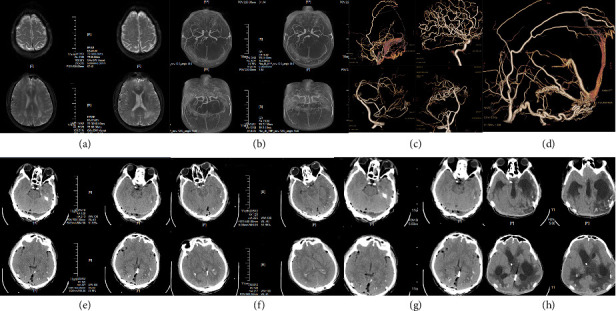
The patient, a 54-year-old male, was admitted to the hospital with a chief complaint of “gradually worsening low mood over the past year, with significant exacerbation in the last two months.” (a, b) Brain imaging revealed multiple abnormal vascular structures, including arteriovenous fistulas, accompanied by white matter edema, congestion, and demyelinating changes. (c, d) Cerebral angiography confirmed the presence of dural arteriovenous fistulas involving the bilateral transverse sinuses, sigmoid sinuses, and the torcular herophili (confluence of sinuses). (e) On the first postoperative day, the patient remained in a drowsy state of consciousness; a follow-up cranial CT scan showed no evidence of intracranial hemorrhage or cerebral infarction. (f) On the first postoperative night, the patient experienced a sudden loss of consciousness, and an emergency cranial CT scan revealed diffuse cerebral edema, more pronounced than preoperatively. (g) The patient subsequently underwent bilateral frontotemporal, parietal, and cerebellar decompressive craniectomy, and postoperative cranial imaging demonstrated improvement in cerebral edema compared to preintervention scans. (h) Despite these interventions, the patient's overall prognosis remained unfavorable.
